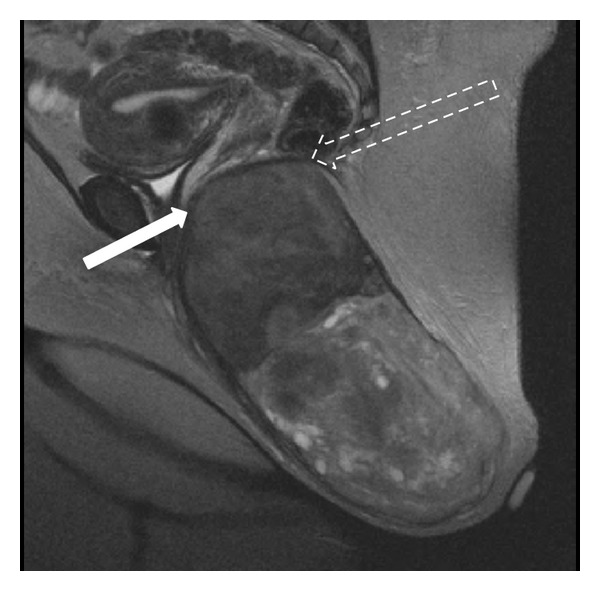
MRI sagittal plane. 23 cm (craniocaudal) mass exhibits midrange T2 weighted signal superiorly with diminished T2 weighted signal at its inferior position. White arrow showing the mass abutting the posterior wall of the vagina. Dashed arrow showing the rectum wall.
